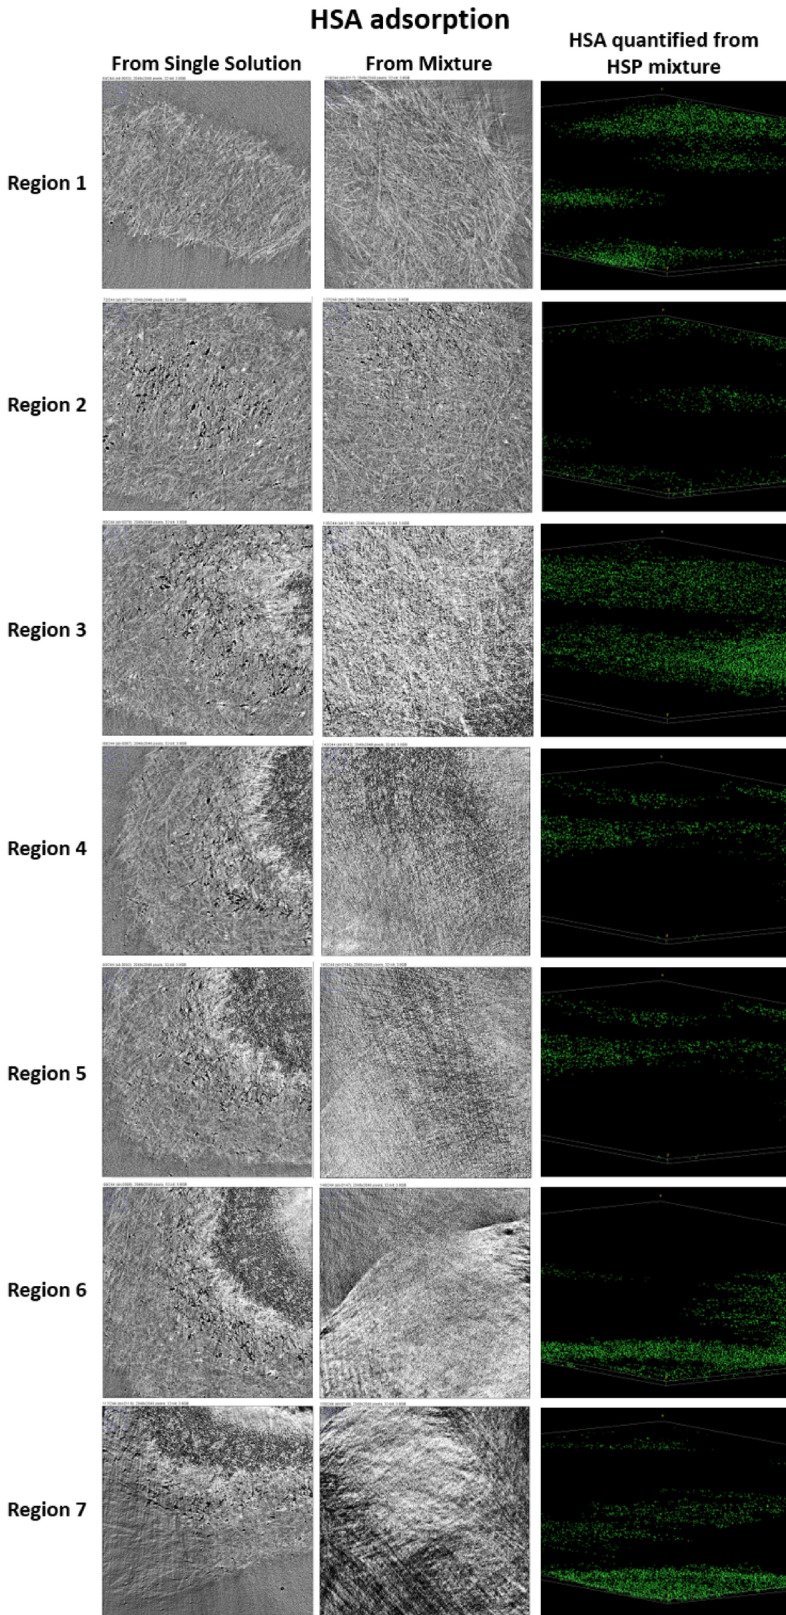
Converted radiographs of the PES membrane with adsorbed HSA from its single solution and HSP, and visualization of the HSA distribution across the membrane thickness.
